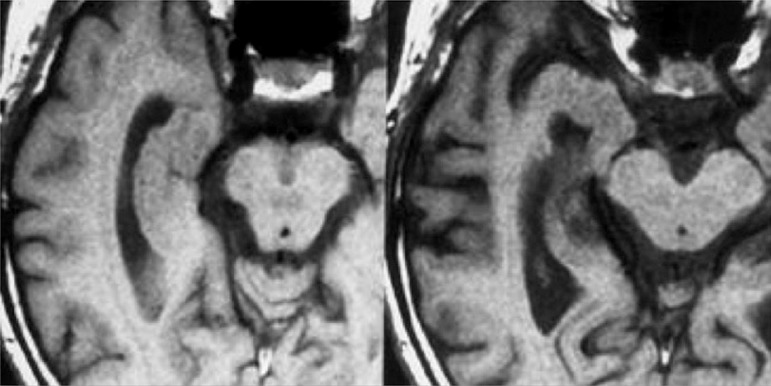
Example of structural MRI showing atrophy of the hippocampus. The right
